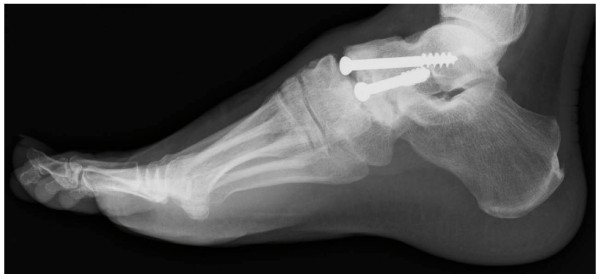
Lateral radiograph at one year after the operation showed completely united talonavicular joint.
